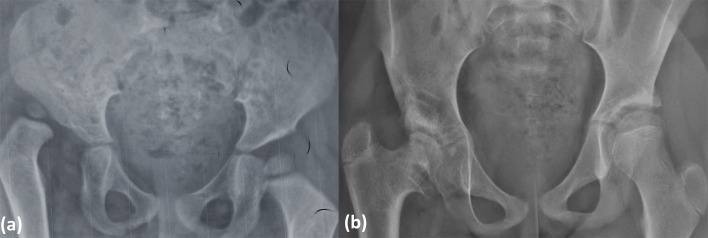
(a) Preoperative radiograph of a girl 2.5 years old of age with right DDH; (b) radiograph at the age of 11 (8.5 years follow-up after open reduction combined to Dega pelvic osteotomy) showing AVN type III of Kalamchi and Macewen with coxa vara.
